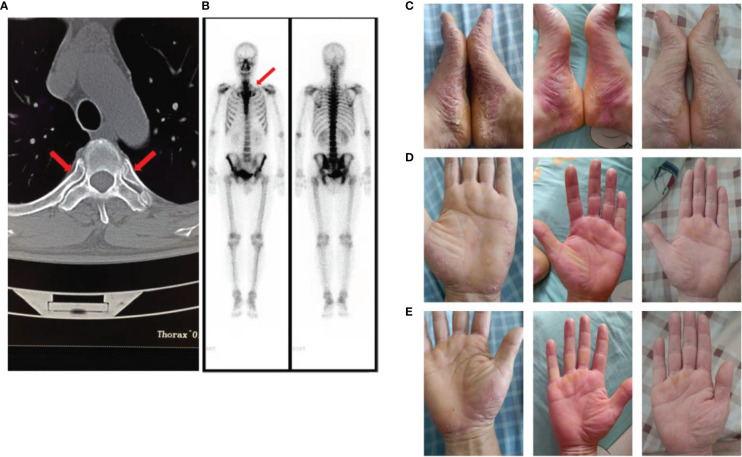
(A) The computed tomography image showed inflammatory changes of the breastbone; (B) The “bull’s head sign” on bony scintigraphy; (C) Palmoplantar pustulosis (PPP) on the patient’s palms and feet before using tofacitinib; (D, E). PPP on the patient’s palms and feet after using tofacitinib for one month and two months.
